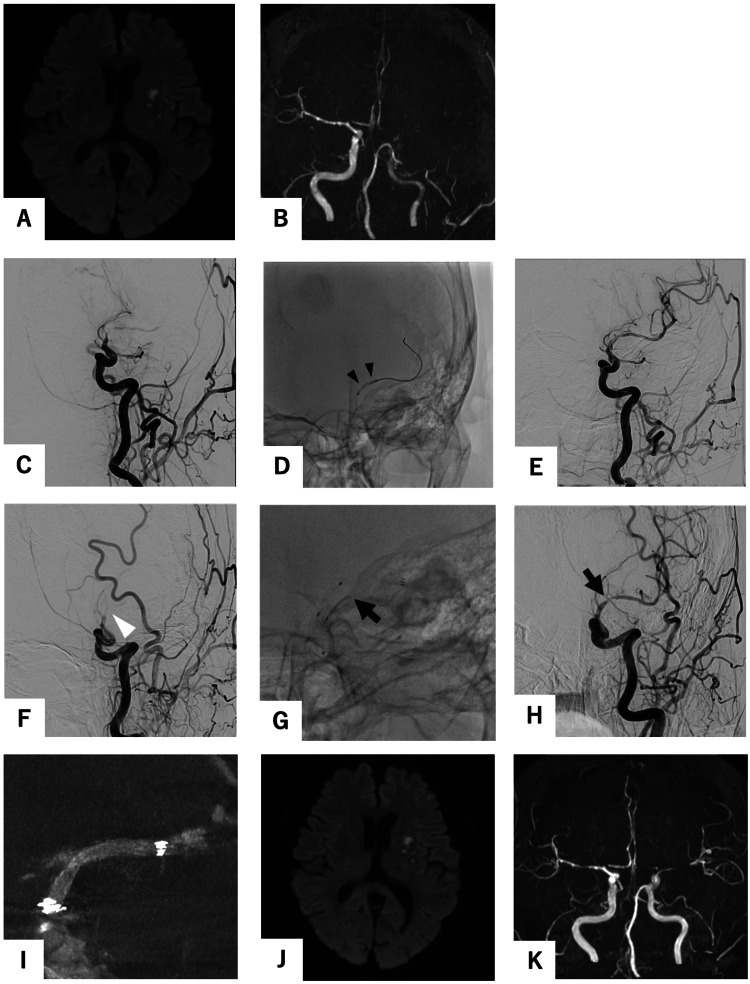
Endovascular surgery and postoperative magnetic resonance imaging (MRI). (A) Diffusion-weighted imaging (DWI) at the time of deterioration showed no new infraction area compared to the previous one. (B) Magnetic resonance angiography (MRA) showed a re-occlusion of the left ICA. (C) As in the first cerebral angiogram, common carotid artery angiogram (CCAG) revealed a severe stenosis of the left ICA, accompanied by a markedly delayed blood flow to the left middle cerebral artery region. (D) A Gateway MR 2.0 × 12 mm (Stryker, Kalamazoo, MI, USA) (black arrowhead) was introduced into the stenotic lesion. Despite performing percutaneous transluminal angioplasty (PTA) (E), restenosis was observed immediately (white arrowhead). (F) We performed PTA using Gateway MR 2.0 × 12 mm at the stenotic lesion (black arrowhead) after PTA allowed spontaneous recanalization. Soon after, CCAG showed restenosis at balloon expansion segment (white arrowhead). (G) The Neuroform Atlas 3.0 × 21 mm (Stryker, Kalamazoo, MI, USA) (black arrow) was placed within the stenosis. (H) Subsequent to the stenting procedure, the CCAG demonstrated the existence of recanalization (black arrow), exhibiting no indications of delayed blood flow. (I) Cone-beam computed tomography showed that the Neuroform Atlas was well adhered to the vessel wall and the stenosis was dilated. (J) Postoperative DWI showed no enlargement of the infraction area. (K) Postoperative MRA showed more improved intensity in the peripheral left middle cerebral artery.
